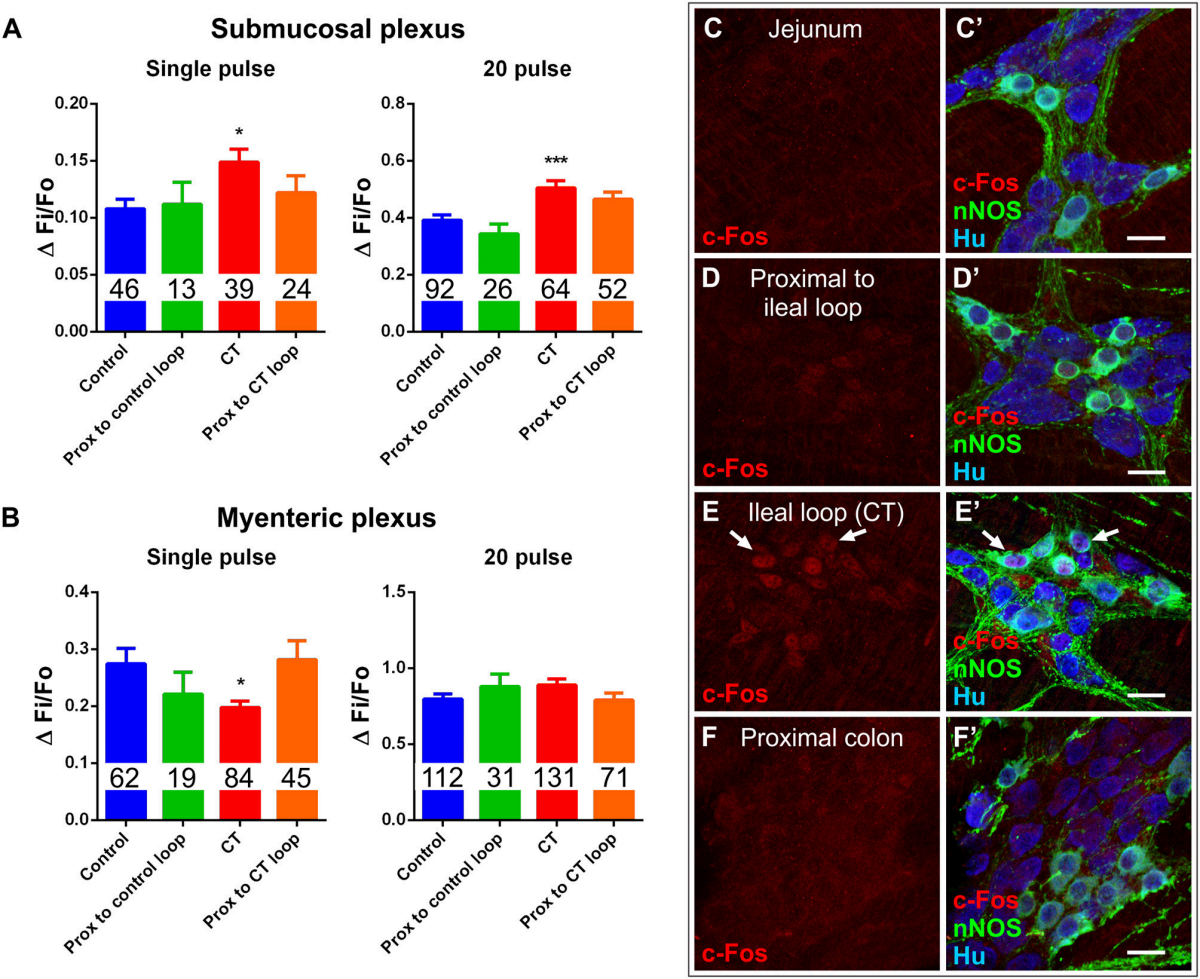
Effects of CT-exposure were confined to the ileal loop. (A) [Ca^2+^]_i_ responses of submucosal neurons to electrical stimulation (single pulse and 20 pulse) in segments of ileum proximal to the control loop or proximal to the CT-treated loop did not differ from controls (^*^*P* < 0.05, ^*^*P* < 0.0001 vs. control). (B) Response amplitudes (ΔFi/F0) of myenteric neurons to electrical stimulation (single pulse and 20 pulse) in segments of ileum proximal to the control loop or proximal to the CT-treated loop also did not differ from controls (^*^*P* < 0.05 vs. control). Number of neurons examined are displayed within the bars. Confocal images of myenteric ganglia from jejunum (C,C′), a segment proximal to the ileal loop (D,D′), proximal colon (F,F′), and the CT-treated ileal loop (E,E′), labeled for c-Fos (red), nNOS (green), and the neuronal marker Hu (blue). Arrows indicate neurons (Hu^+^) displaying colocalization of c-Fos- and nNOS-immunoreactivity. Nuclear c-Fos labeling was confined to the CT-treated ileal loop and was not observed in other intestinal regions. Scale bars = 20 μm.
